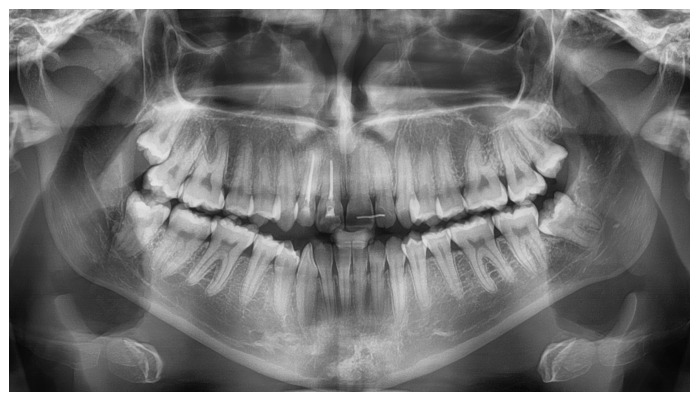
Panoramic radiograph 4 years after treatment completion.
